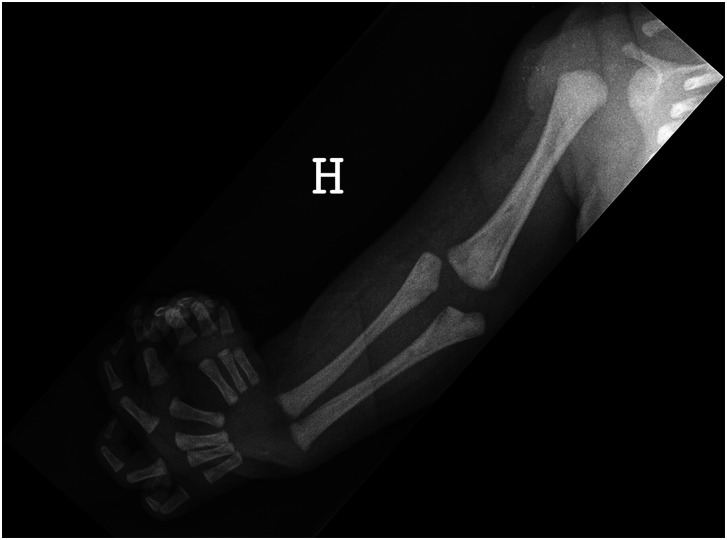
Radiograph of Case 23 shows a normal gleno-humeral joint, a dysplastic broad distal humerus, an absent radius, duplicated ulna and polydactyly.
